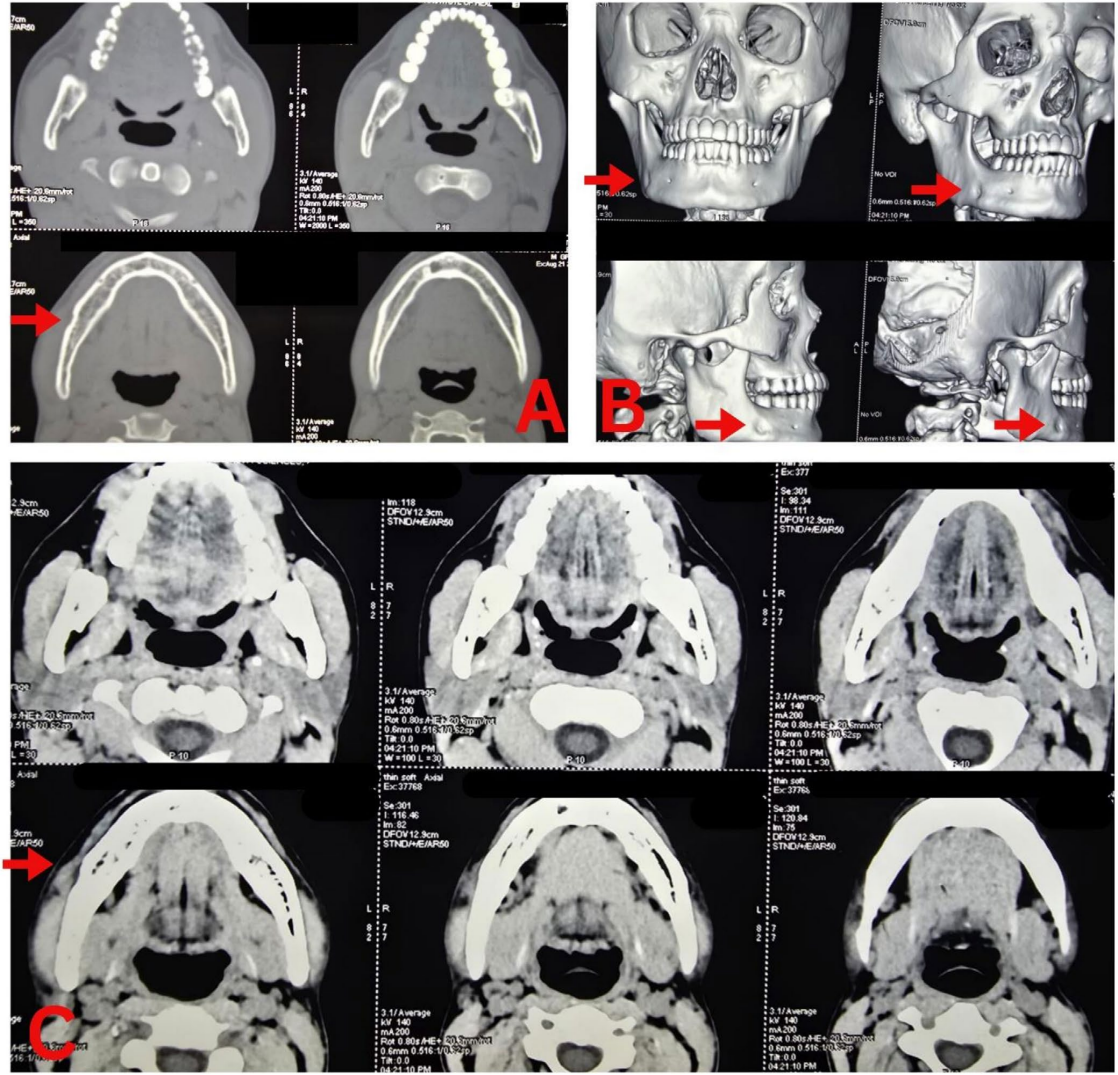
CT images showing (A) bony window, (B) 3D reconstruction showing scalloping of underlying bone due to lesion, (C) soft tissue window (axial section). The area of interest is indicated by a red arrowhead.
